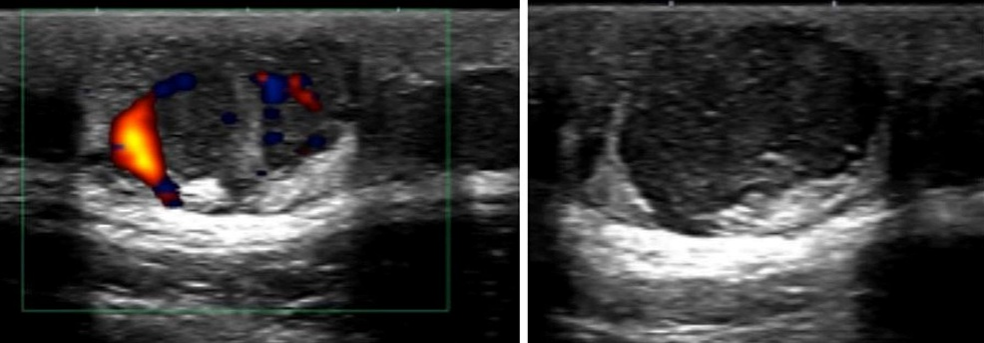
Sonographic image of the dermatofibrosarcoma protuberans lesion showing heterogeneous echogenicity and vascularity of the mass, as evidenced by Doppler flow.
